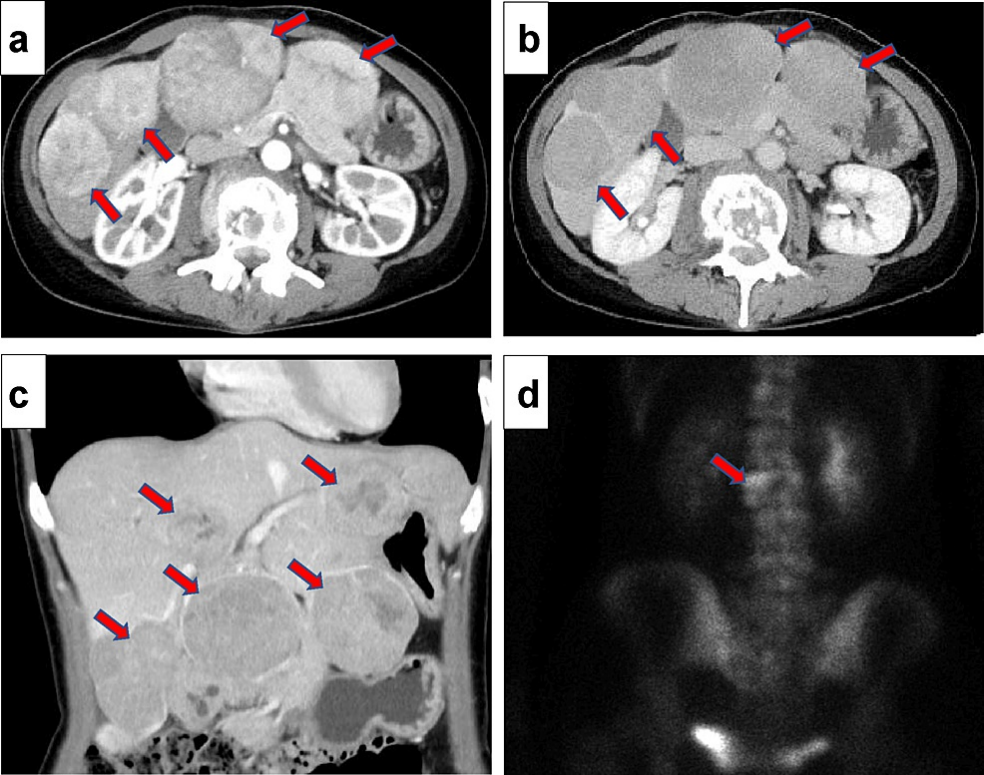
Diagnostic imaging of hepatocellular carcinoma: contrast-enhanced CT and bone scintigraphy findings a-c) Contrast-enhanced computed tomography shows that there are multiple tumors (red arrows) of up to 65 mm diameter in both lobes of the liver, which is a typical finding of hepatocellular carcinoma, with tumor enhancement in the early phase and washout in the late phase. d) Bone scintigraphy shows metastatic lesions (red arrows) in the L3 vertebral body.
